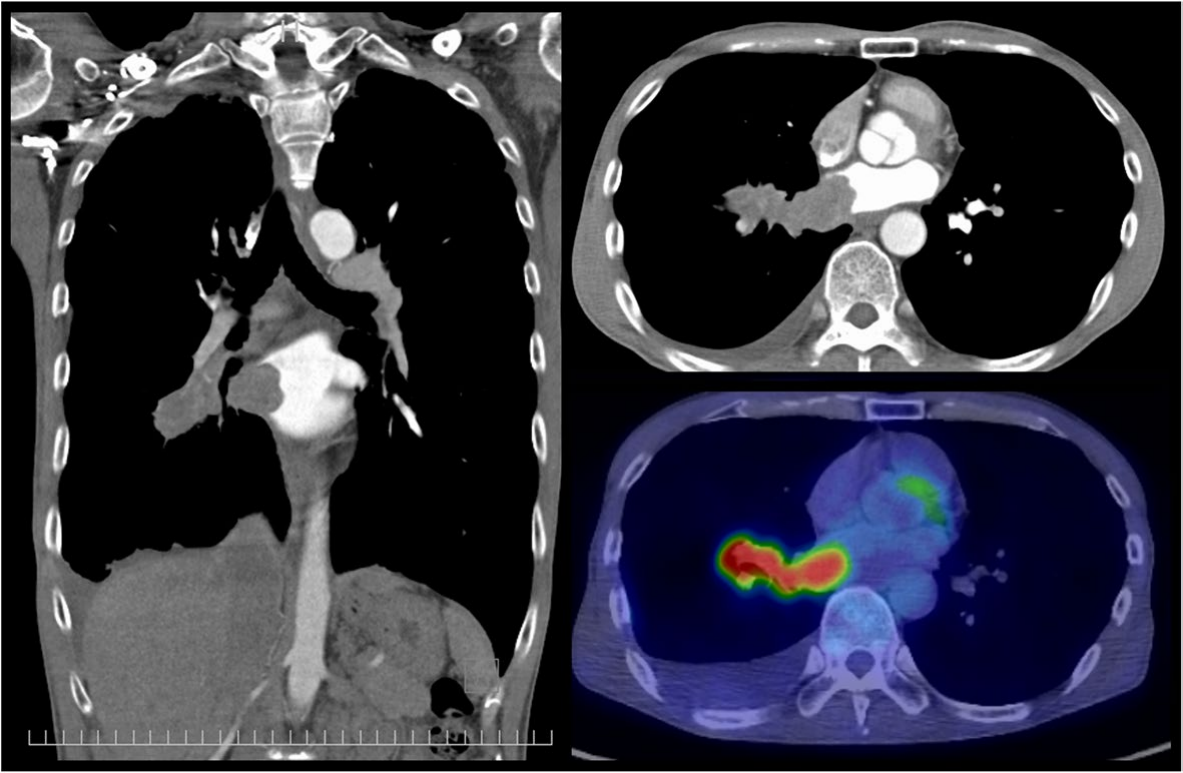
Computed tomography and positron emission tomography before osimertinib treatment. Computed tomography at the initial diagnosis (coronal section [left] and transverse section [right upper]) showed a 3.4-cm tumor originating from the right lower lobe and enlarged hilar nodes that invaded into the left atrium through the lower pulmonary vein. Positron emission tomography revealed a strong glucose uptake with the maximum standardized uptake value of 10.3 (right lower)
